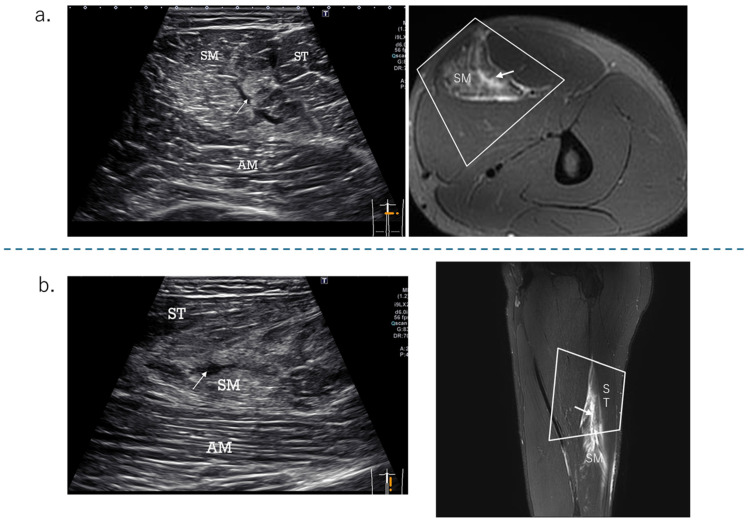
The US findings of the SM proximal musculotendinous junction tear. (a) Ultrasound short-axis image: a tear and hematoma (white arrow) are seen at the proximal MTJ of the SM. (b) Long-axis image: a tear and hematoma (white arrow) and edema are seen between the ST and AM (adductor magnus). It is easier to understand if you refer to the sagittal MRI image.
